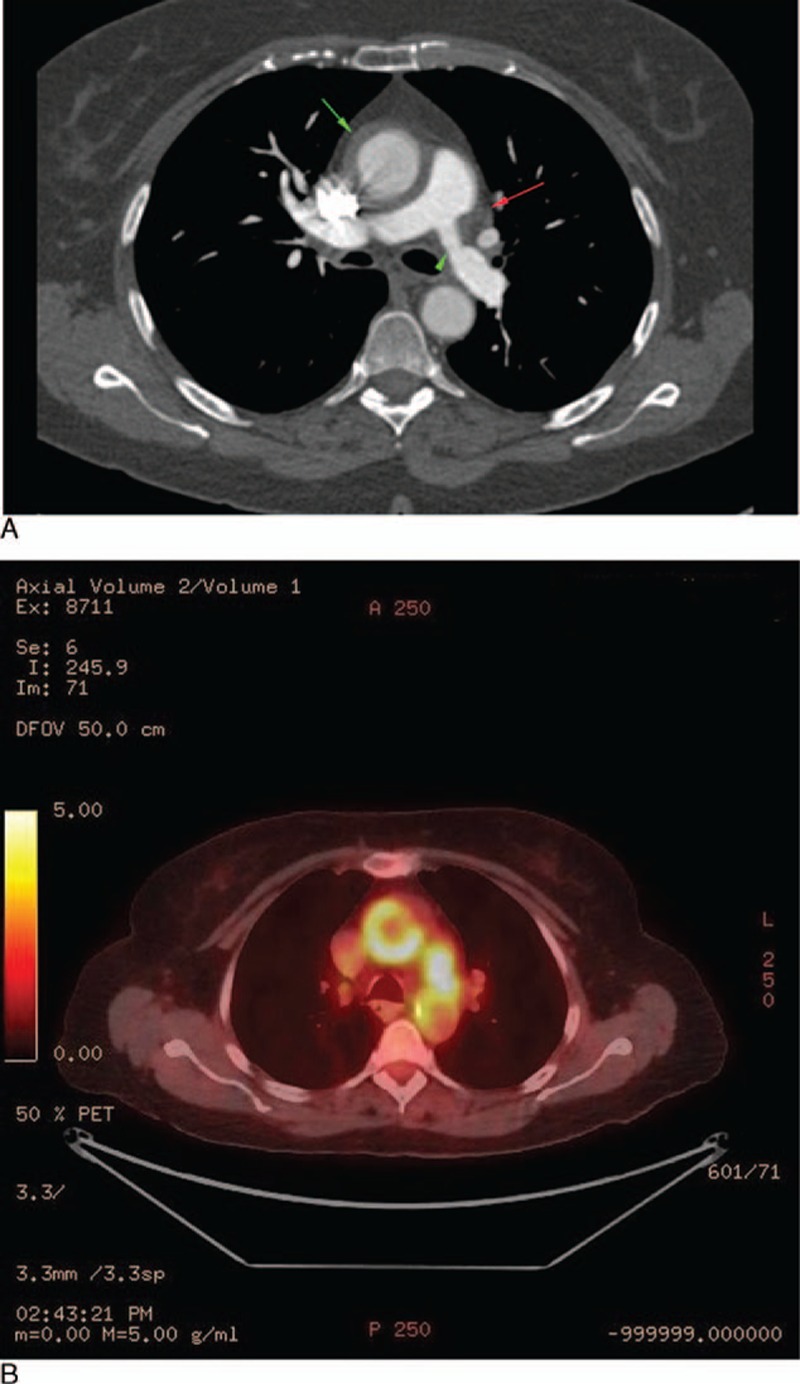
(A) Cross-sectional CT chest with contrast displaying a soft tissue mass compressing the left pulmonary artery (red arrow) with resulting pulmonary arterial stenosis (green arrow head). Also seen is circumferential thickening and contrast enhancement of the ascending thoracic aorta (green arrow). (B) PET-CT showing FDG avidity of both the soft tissue mass around the pulmonary artery as well as the ascending thoracic aortic wall.
